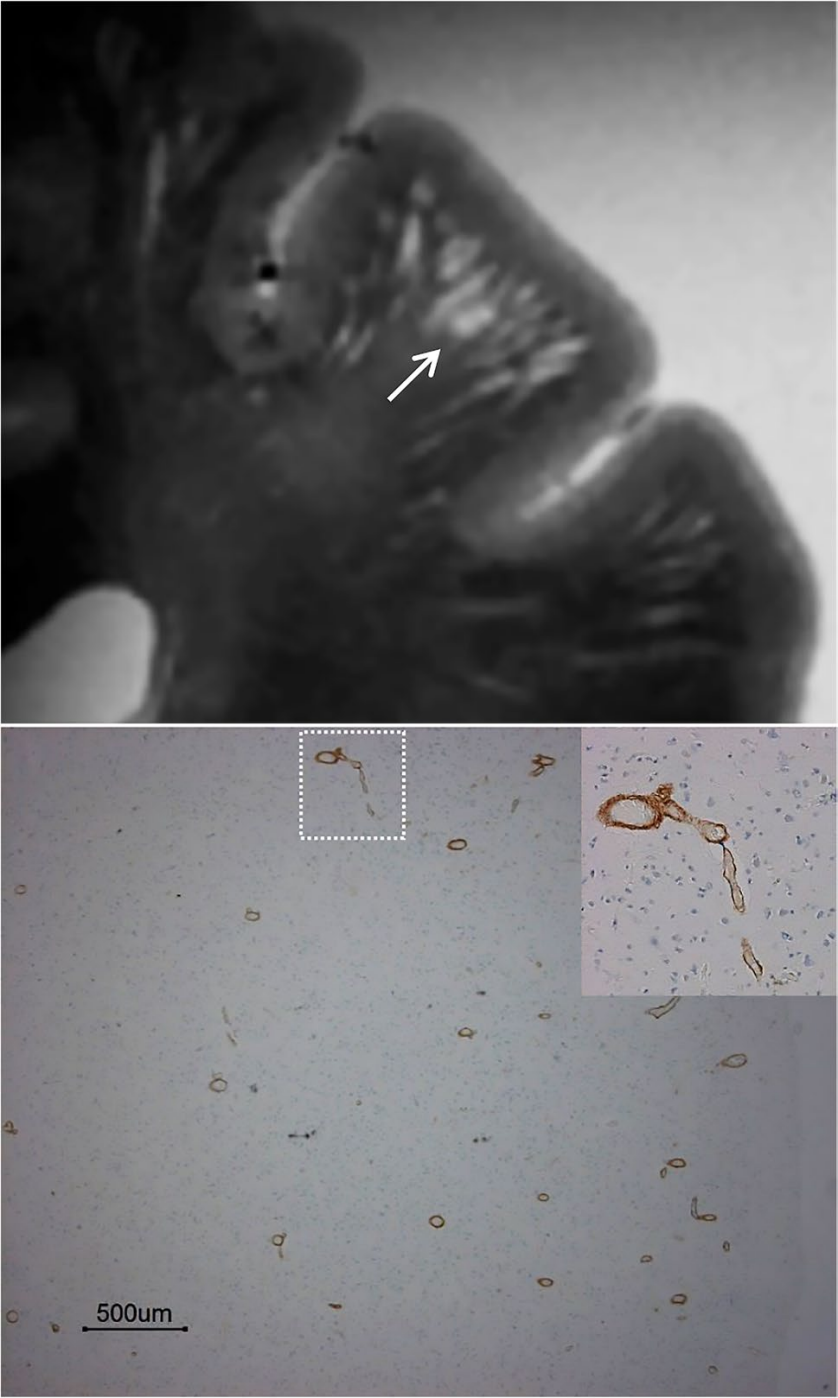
Enlarged juxtacortical perivascular spaces (PVS). Post-mortem T2-weighted magnetic resonance image acquired at 7 T (top) showing sharply demarcated juxtacortical hyperintensities corresponding to “many severely enlarged PVS” (arrow) in a patient with pathologically confirmed cerebral amyloid angiopathy (bottom). Please note the absence of PVS at the cortical level. Modified and reproduced with permission from the Publisher (SAGE) and the authors: van Veluw SJ, Biessels GJ, Bouvy WH, Spliet WG, Zwanenburg JJ, Luijten PR, Macklin EA, Rozemuller AJ, Gurol ME, Greenberg SM, Viswanathan A, Martinez-Ramirez S. Cerebral amyloid angiopathy severity is linked to dilation of juxtacortical perivascular spaces. *Journal of Cerebral Blood Flow and Metabolism*. 2016; 36(3):576–580 [91]
